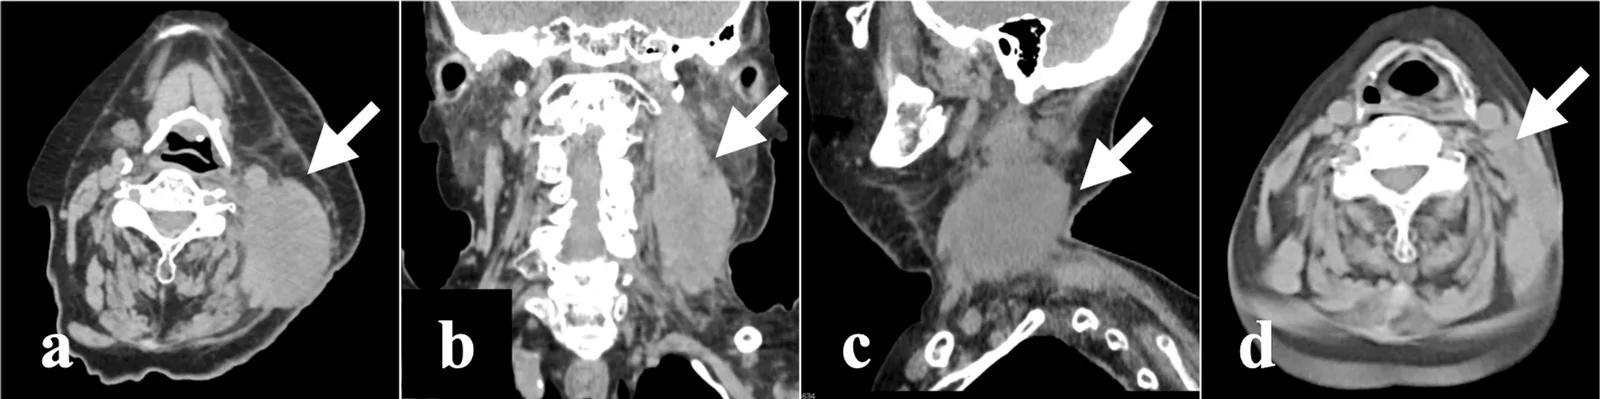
Non-contrast CT images of the patient's neck The images are showing the left cervical lymph node metastasis following SBRT: (a–c) non-contrast CT obtained on day 7 (the scheduled third fraction), showing initial tumor regression (white arrow). (a) Axial, (b) coronal, and (c) sagittal views. (d) Axial non-contrast CT obtained 37 days after SBRT, showing further tumor regression (white arrow). CT; computed tomography, SBRT; stereotactic body radiotherapy.
